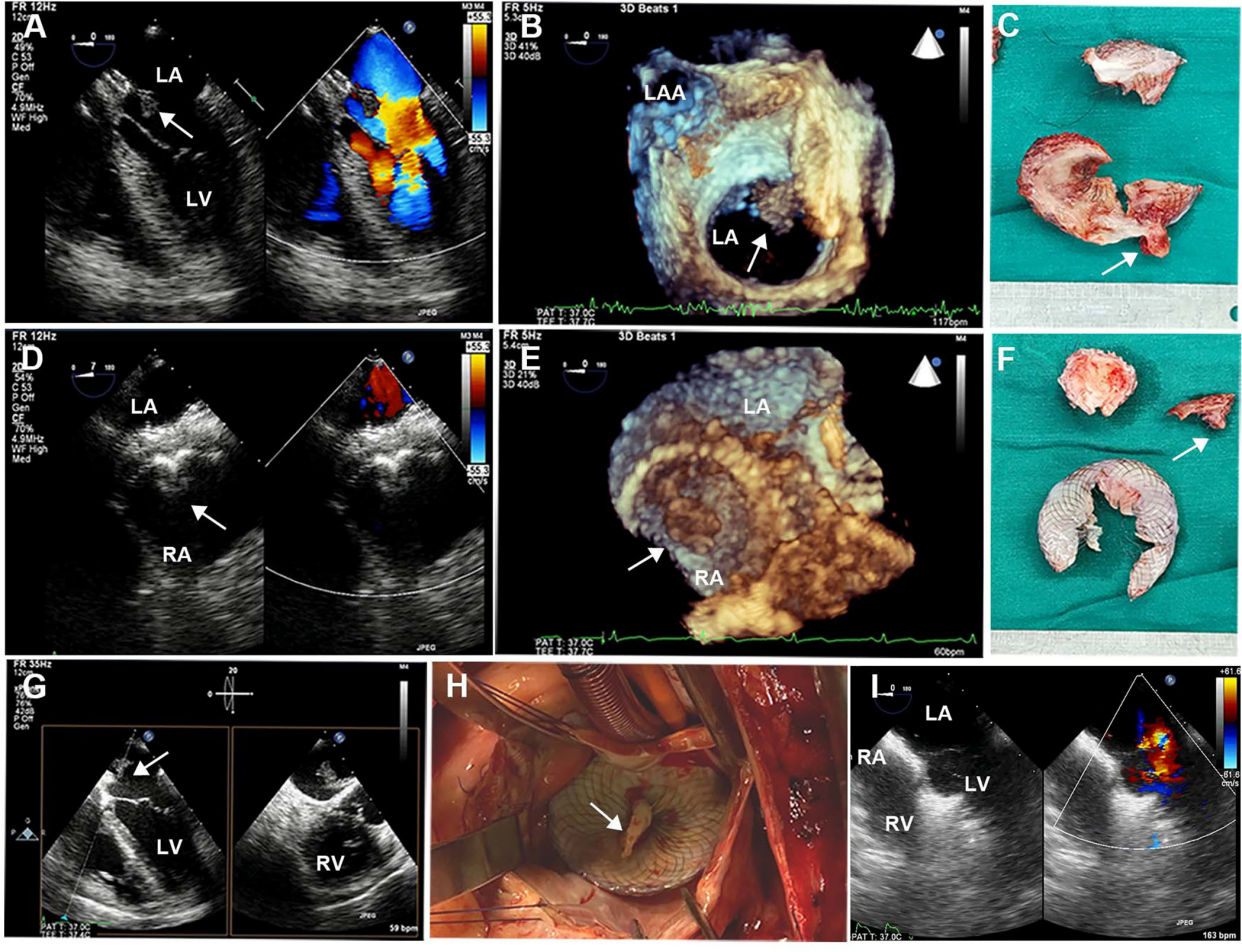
Intraoperative TEE and surgical images. (A,D) 2D-TEE color Doppler showed an ASD device with a double-sided thrombus. (B,E) 3D-TEE demonstrated a fan-like left-atrial thrombus attached to the device and a short rod-like right-atrial thrombus attached to the device. (C,F) Surgical resection of ASD device fragments with bilateral thrombus. (G) Biplanar TEE showed a giant thrombus floating in the left atrium. (H) Left atriotomy showed a white thrombus attached to the device. (I) Postoperative TEE showed continuous atrial septum without shunt and thrombus. TEE, transesophageal echocardiography; ASD, atrial septal defect. White arrows represent thrombus.
